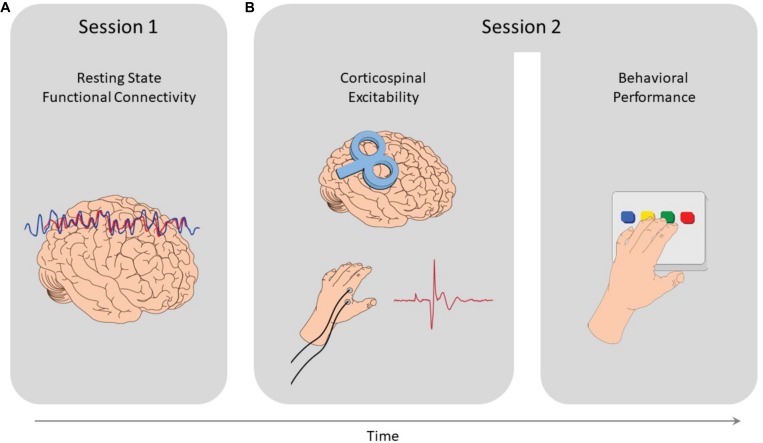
Study design. (A) In the first session resting-state functional MRI scans were acquired, from which functional connectivity measures where extracted. (B) Then, in the second session, MEPs were induced via single pulse TMS applied over the right M1, and measured from the left FDI muscle to quantify corticospinal excitability. At the end of that session, participants performed a sequential finger tapping task, to measure their motor performance.
